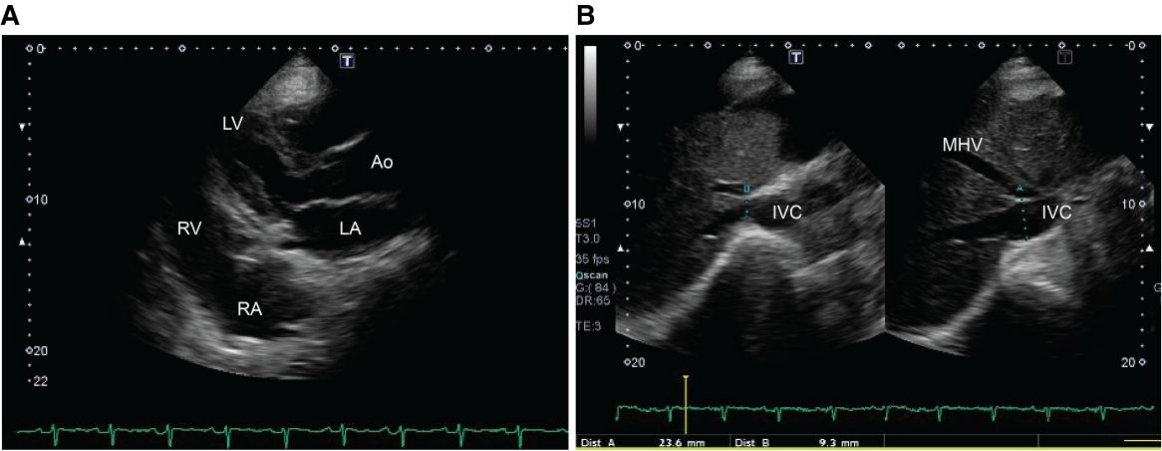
Pre-operative echocardiographic findings. (*A*) Parasternal long axis view reveals the left and right chambers with limited dilation and thickening of the right ventricular epicardium (white arrow). LV, left ventricle; LA, left atrium; RV, right ventricle; Ao, ascending aorta. (*B*) The IVC is dilated to 23.6 mm, with preserved respiratory variability. IVC, inferior vena cava; MHV, middle hepatic vein.
